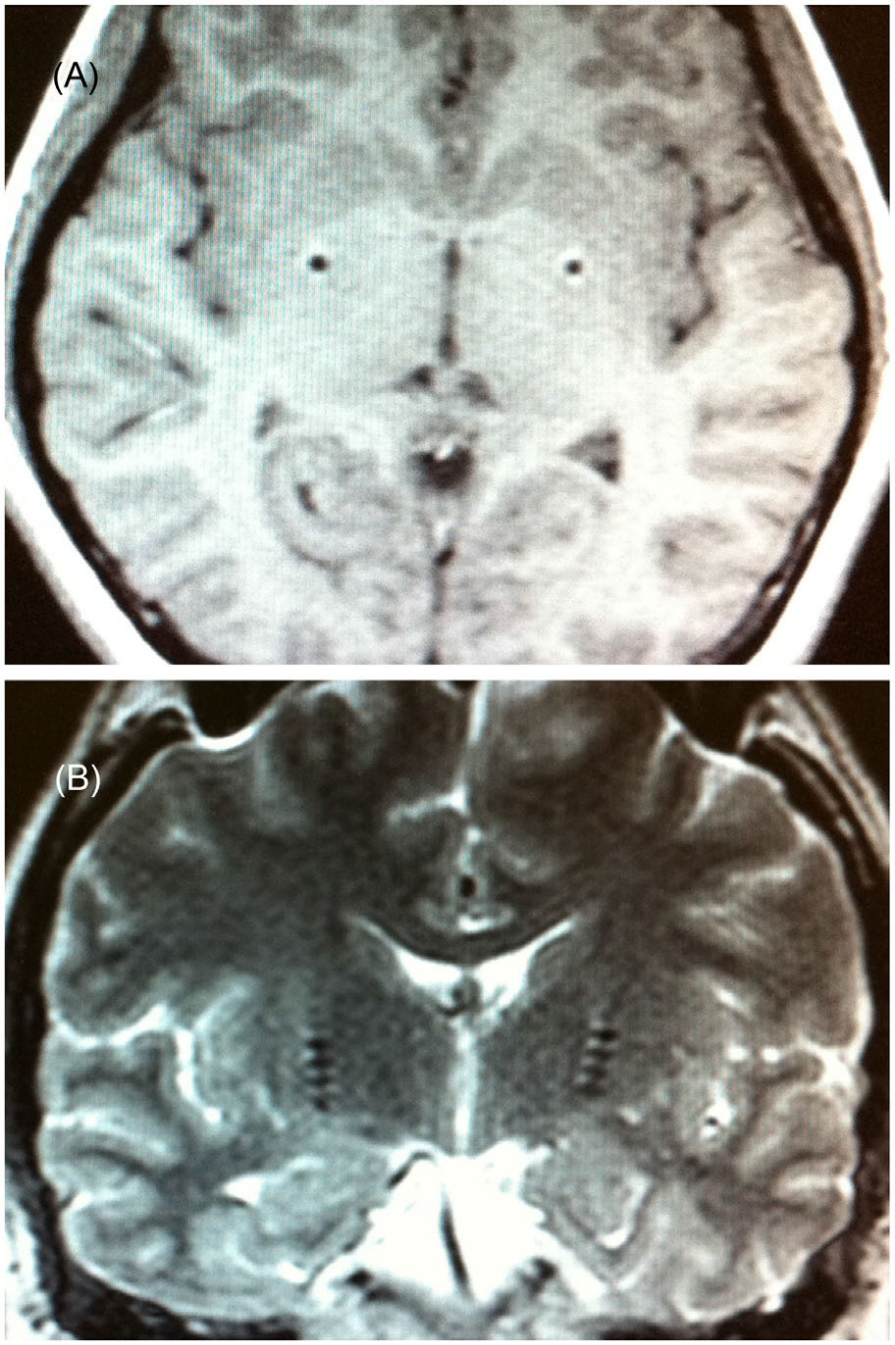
Magnetic resonance imaging (MRI) of axial (a) and coronal (b) slices from one patient showing the trajectory and location of the electrodes implanted bilaterally in the posteroventral globus pallidus interna
